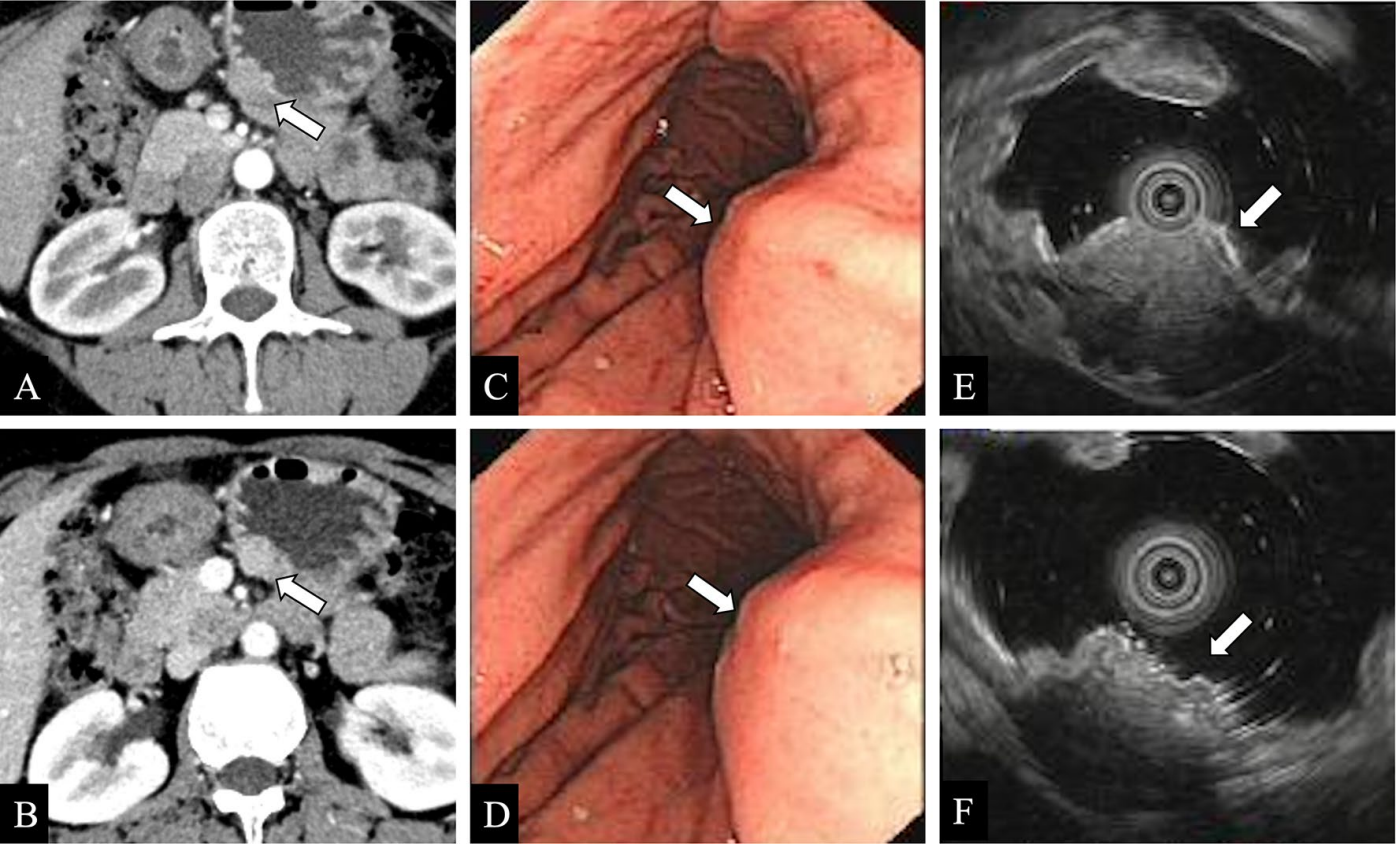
Images demonstrating the gastric body with a submucosa heterotopic pancreas (white arrows) identified within the wall of the stomach. a, b Contrast-enhanced axial CT images in arterial and venous phases. c, d Upper endoscopy images. e, f Endoscopic ultrasonography images
